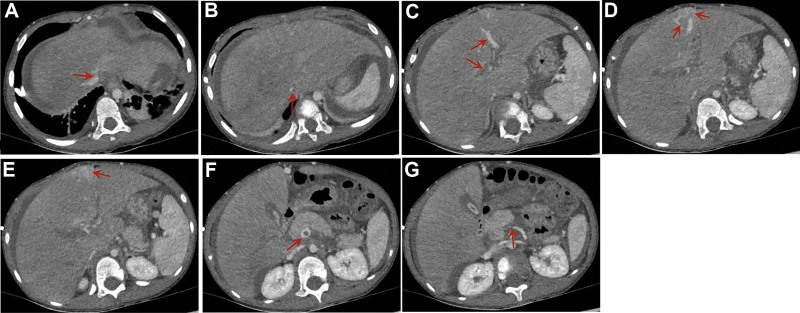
Computed tomography angiography. (A) Unclear display of hepatic veins (red arrow). (B) Hepatomegaly, massive ascites, heterogeneous enhancement of the liver, and stenosis of the hepatic segment of the inferior vena cava (red arrow). (C and D) The right branch of the portal vein was unclear, while the left branch was enlarged, anastomosing with the tortuous and thickened umbilical vein and abdominal wall vein (red arrow). (E) Patchy liver enhancement (red arrow). (F and G) The hypodense shadow of the portal vein and the superior mesenteric vein (red arrow).
